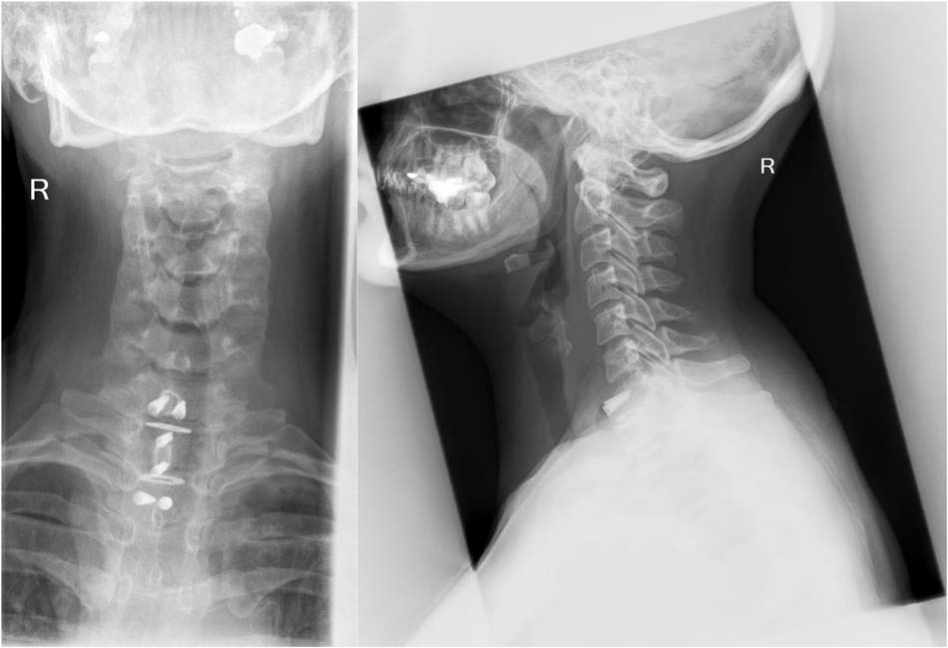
A 3-day postoperative X-ray of VBR and instrumentation in coronal (Right) and sagittal (Left) view.
